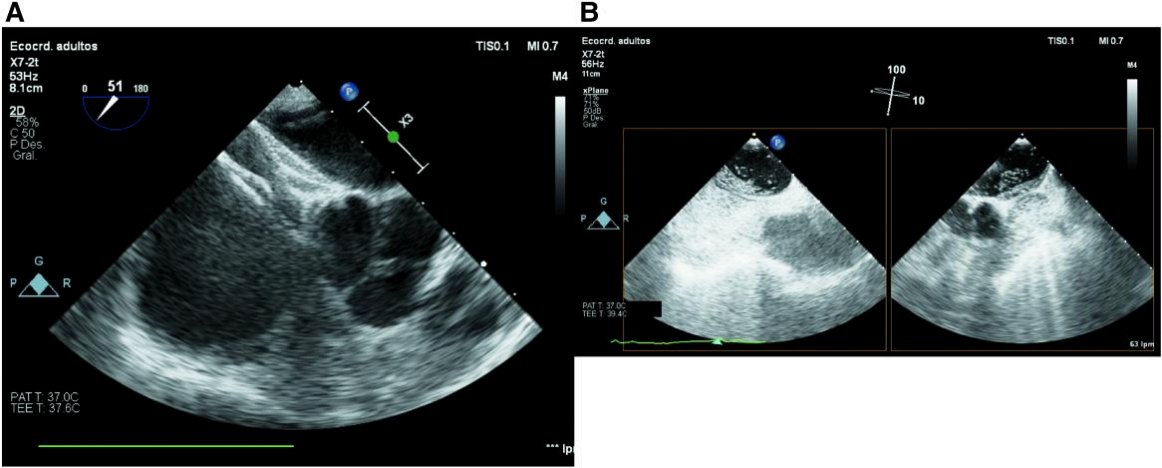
Transoesophageal echocardiography. Amplatzer device position (30 mm) (*A*). Agitated saline contrast test before PFO closure. XPlane view (*B*).
